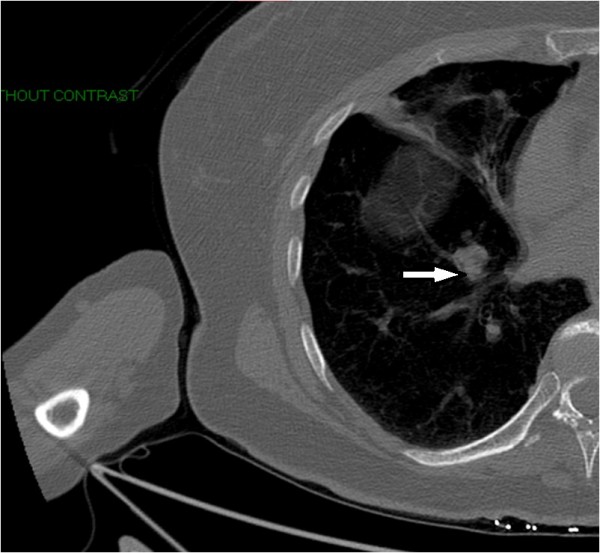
Computed tomography scan of right shoulder without intravenous contrast incidentally shows a rounded 2cm right lower lobe pulmonary mass (white arrow) with no pathologic lesion on right shoulder.
